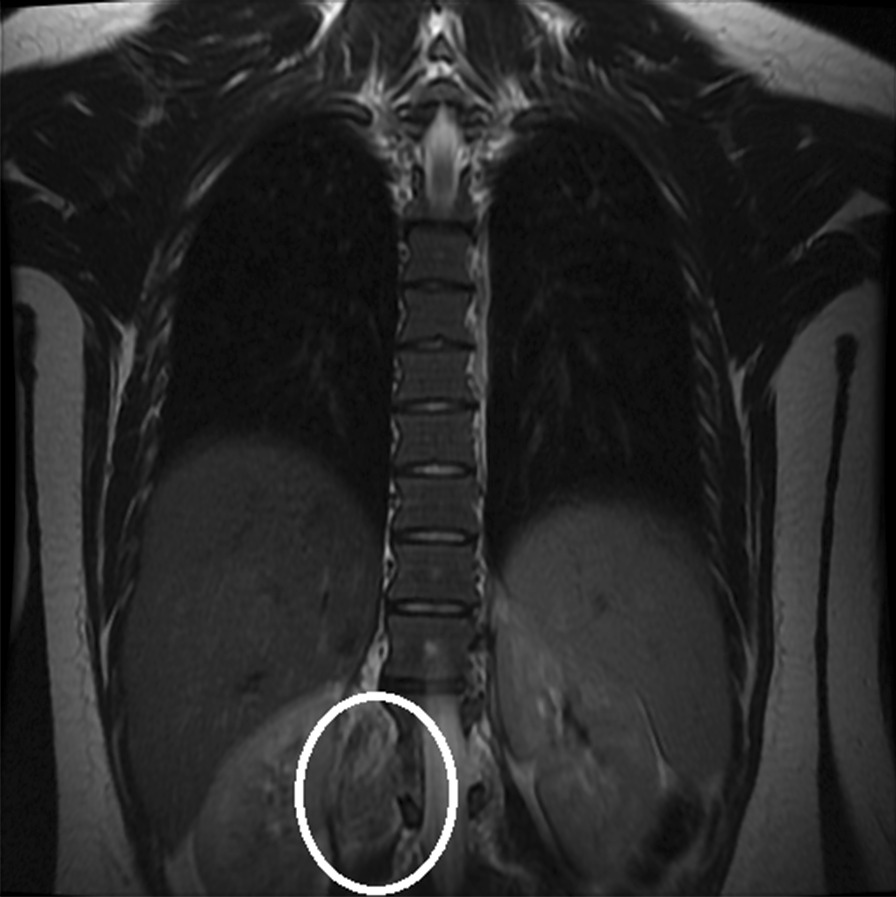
Coronal T2 magnetic resonance imaging. Circle: solid nodular lesion
